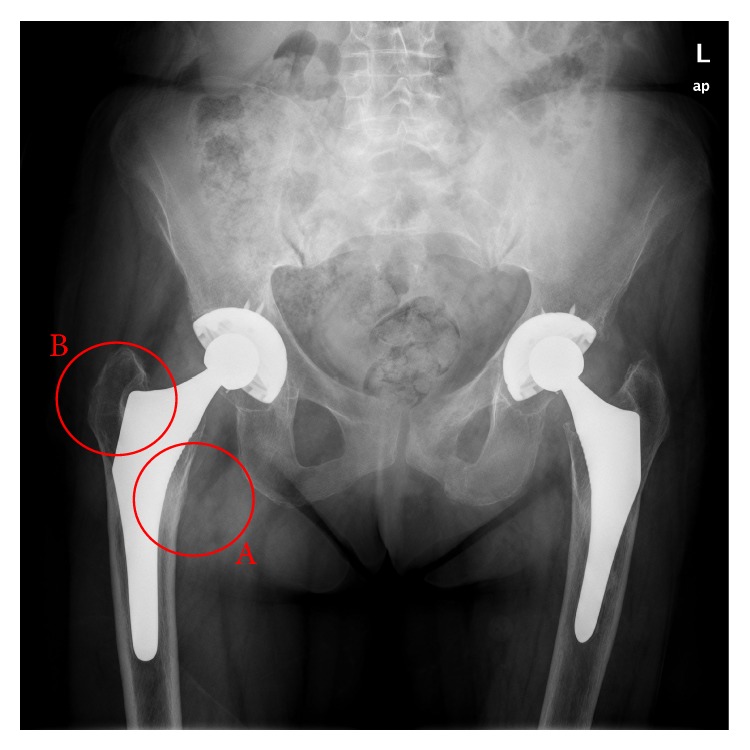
X-ray image of oversized stem in right femur with cancellous bone densification in support area (zone A) and bone resorption (zone B).
